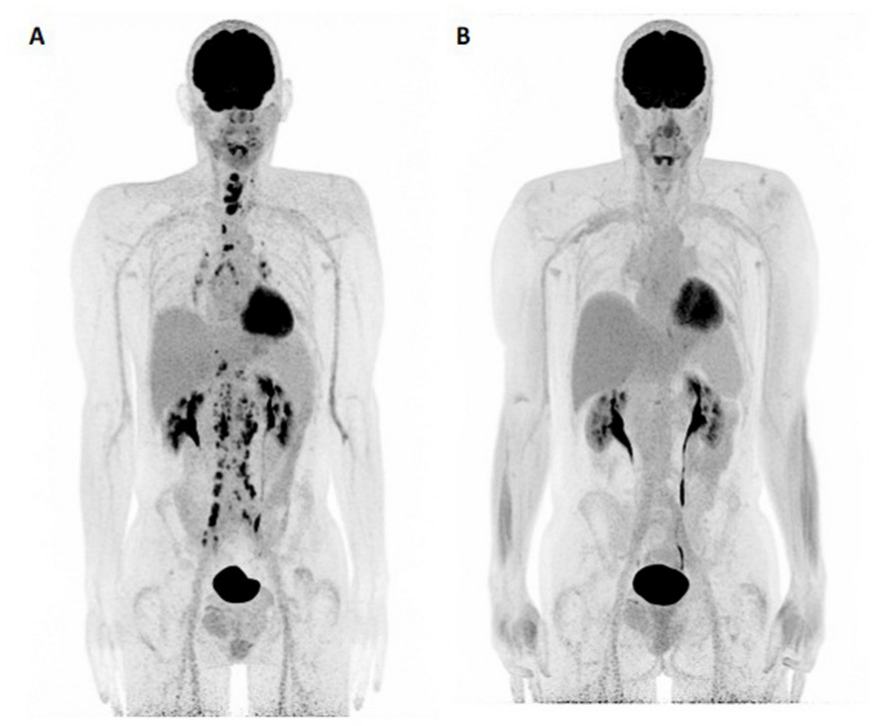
Initial evaluation and follow-up by FDG-PET/CT. (A, B) PET MIP showing all lesions at baseline and their disappearance on follow-up.
